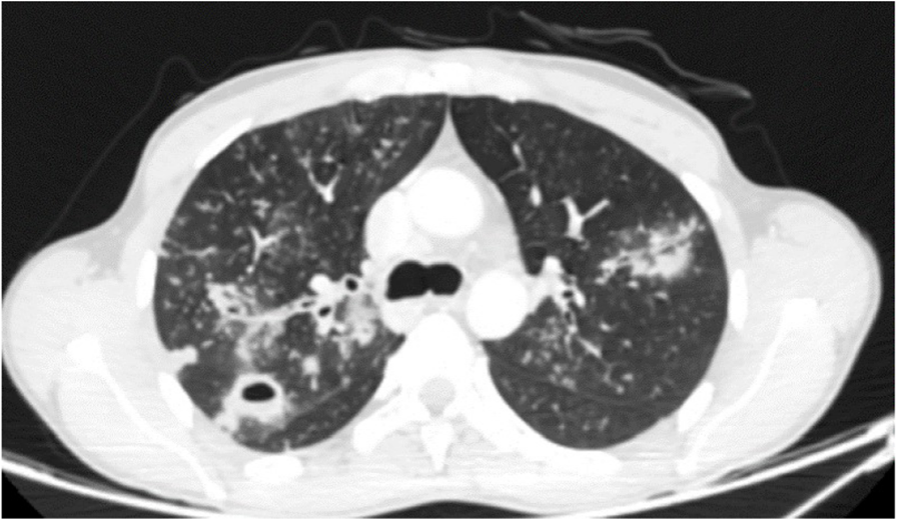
Post primary pattern of tuberculosis: thin section CT shows bilateral tree-in-bud opacities and a cavitary masslike consolidation in the right upper lobe
